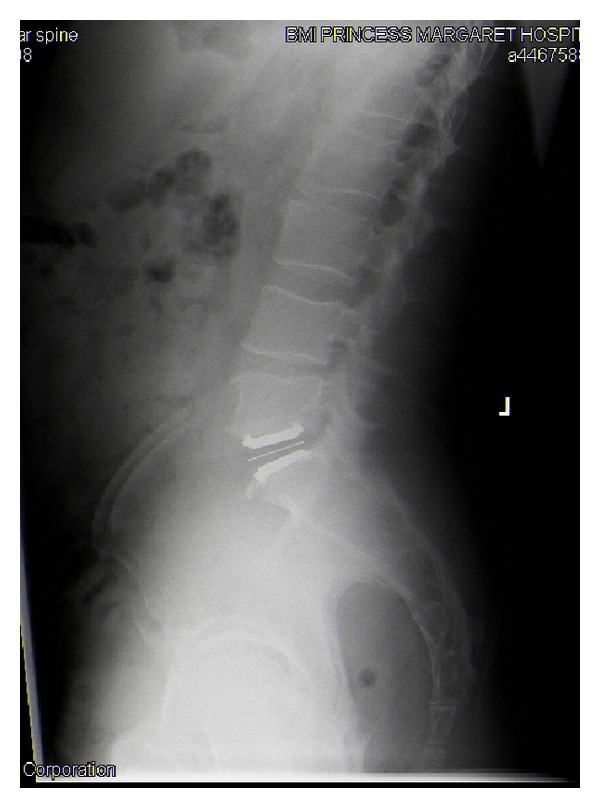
Plain films showing initial placement of total disc replacement.
